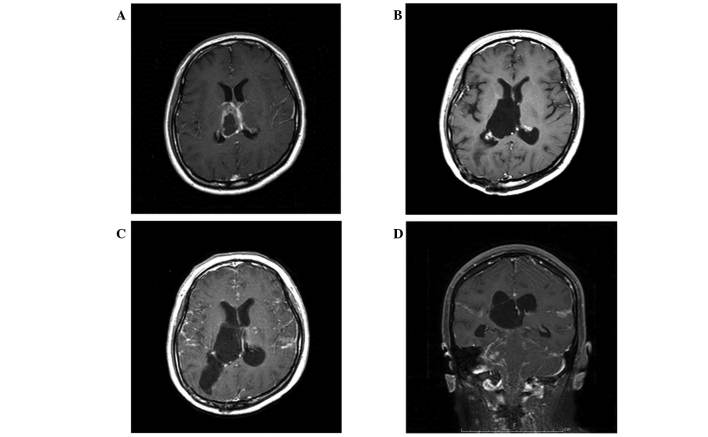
(A) MRI prior to surgery showed a tumor in the right thalamus enhanced with gadolinium diethylenetriamine pentaacetic acid. (B) MRI following maintenance TMZ therapy showed the enhanced lesion in the right thalamus had disappeared without recurrence. (C and D) Recurrent tumor was revealed on MRI; leptomeningeal dissemination was identified in the supra- and infratentorial brain without primary site recurrence. MRI, magnetic resonance imaging; TMZ, temozolamide.
